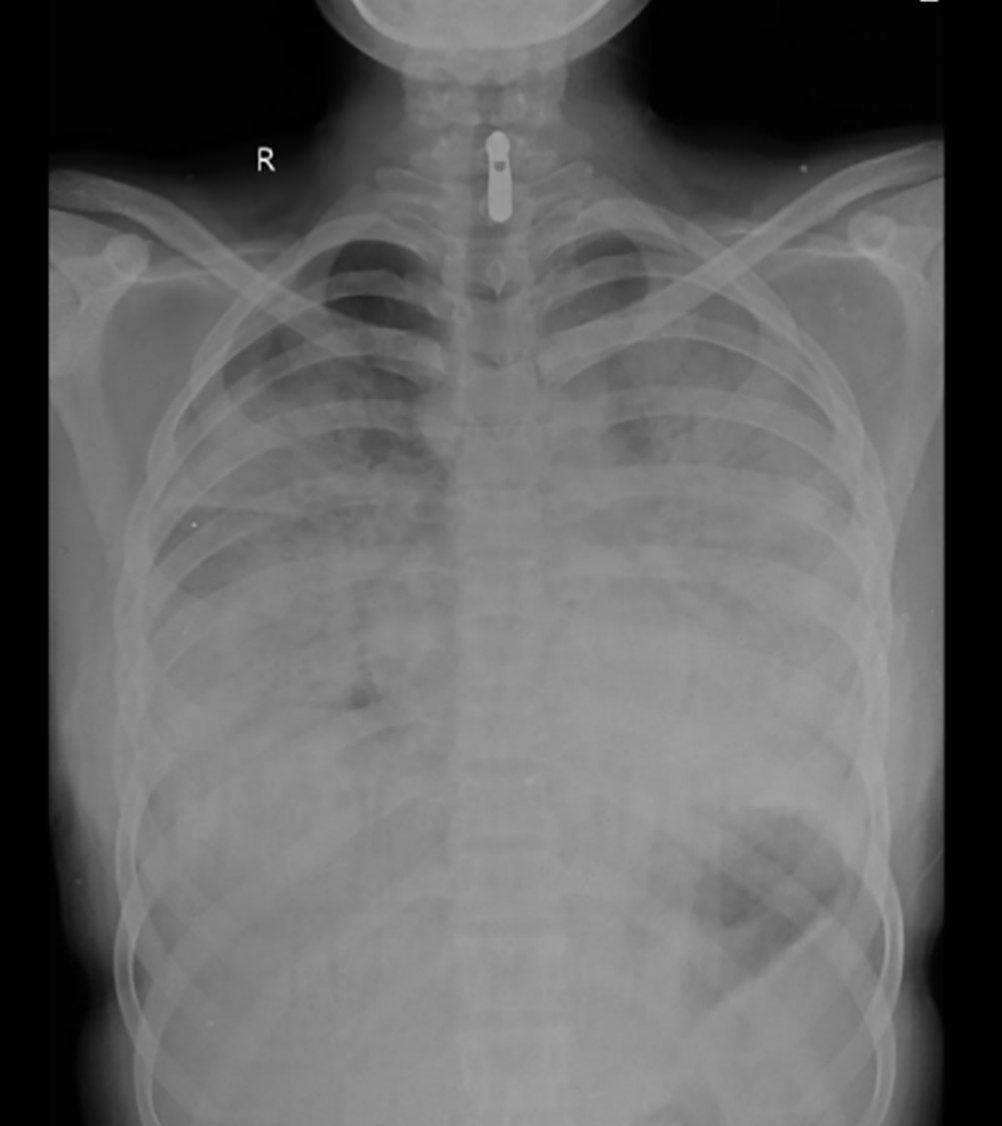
Chest radiograph PA view, day 1.
